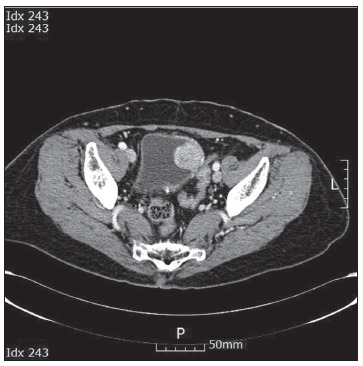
Abdominal computed tomography showing an intraluminal polypoid mass of approximately 3 × 3.2 cm in size with heterogeneous enhancement on the left lateral wall of the urinary bladder.
